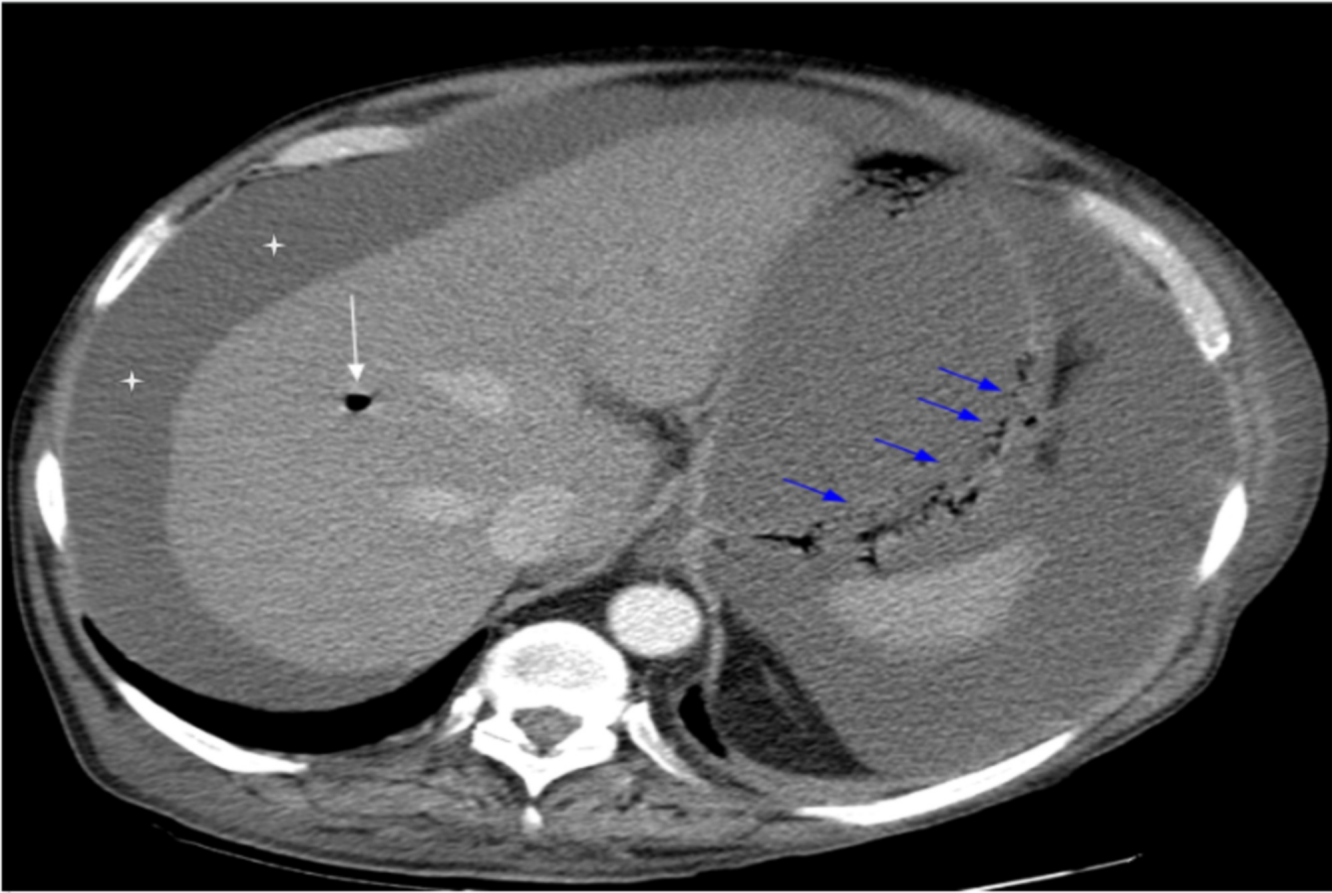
Axial images of the CT abdomen and pelvis with intravenous contrast Axial images of the CT abdomen and pelvis with intravenous contrast reveal a distended stomach with infiltration of the posterior gastric wall with air (blue arrows) consistent with emphysematous gastritis. The focus of air (white arrow) within the liver is suspicious for portal venous gas. A large amount of abdominal ascites (white stars).
